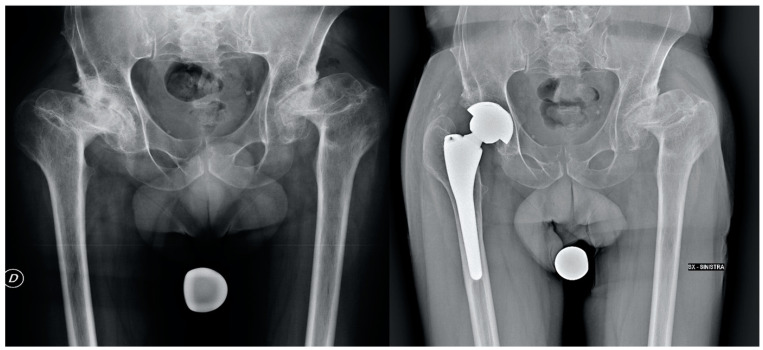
Pre-operative and post-operative X-rays of a patient treated with THA (anatomical stem) for hip dysplasia.
